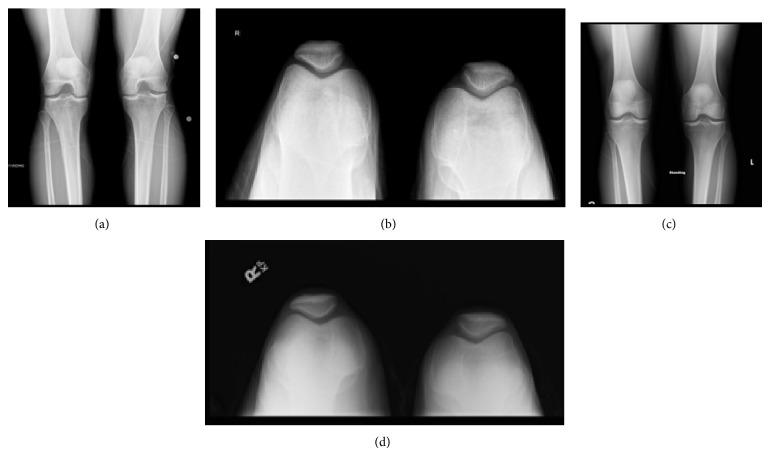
AP and sunrise knee radiographic images of a 29-year-old male patient at (a-b) 8 months and (c-d) 30 months after an acute ACL injury. The images show progression of osteoarthritic changes in a young male with an ACL-deficient knee.
